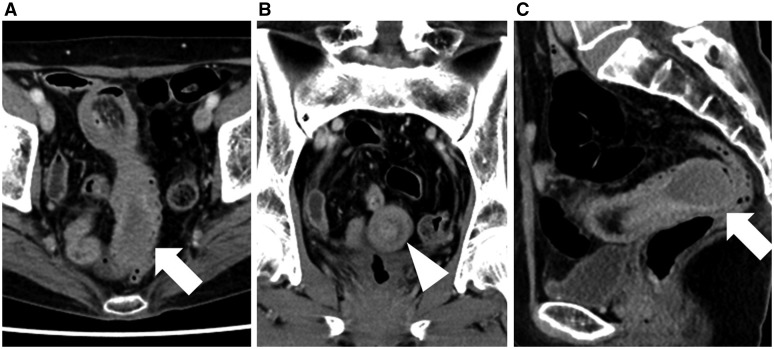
Contrast-enhanced computed tomography. A) Axial; B) Coronal; C) Sagittal images. A target-like structure was revealed with a hypovascular mass (arrows) present at the leading edge. The target-like structure was most evident in (B) (arrowhead).
